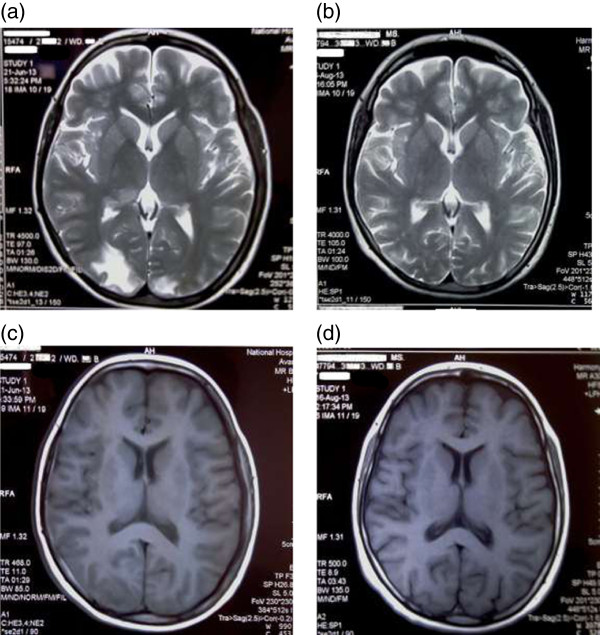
Preliminary and reassessment MRI brain: (a), (c) are preliminary MRI images taken on presentation. (a) - T2 weighted shows high signal intensity and (c) – T1 weighted shows low signal intensity involving the posterior occipital areas with cortical and subcortical regions. (b), (d) are MRI images taken on reassessment showing improvement.
